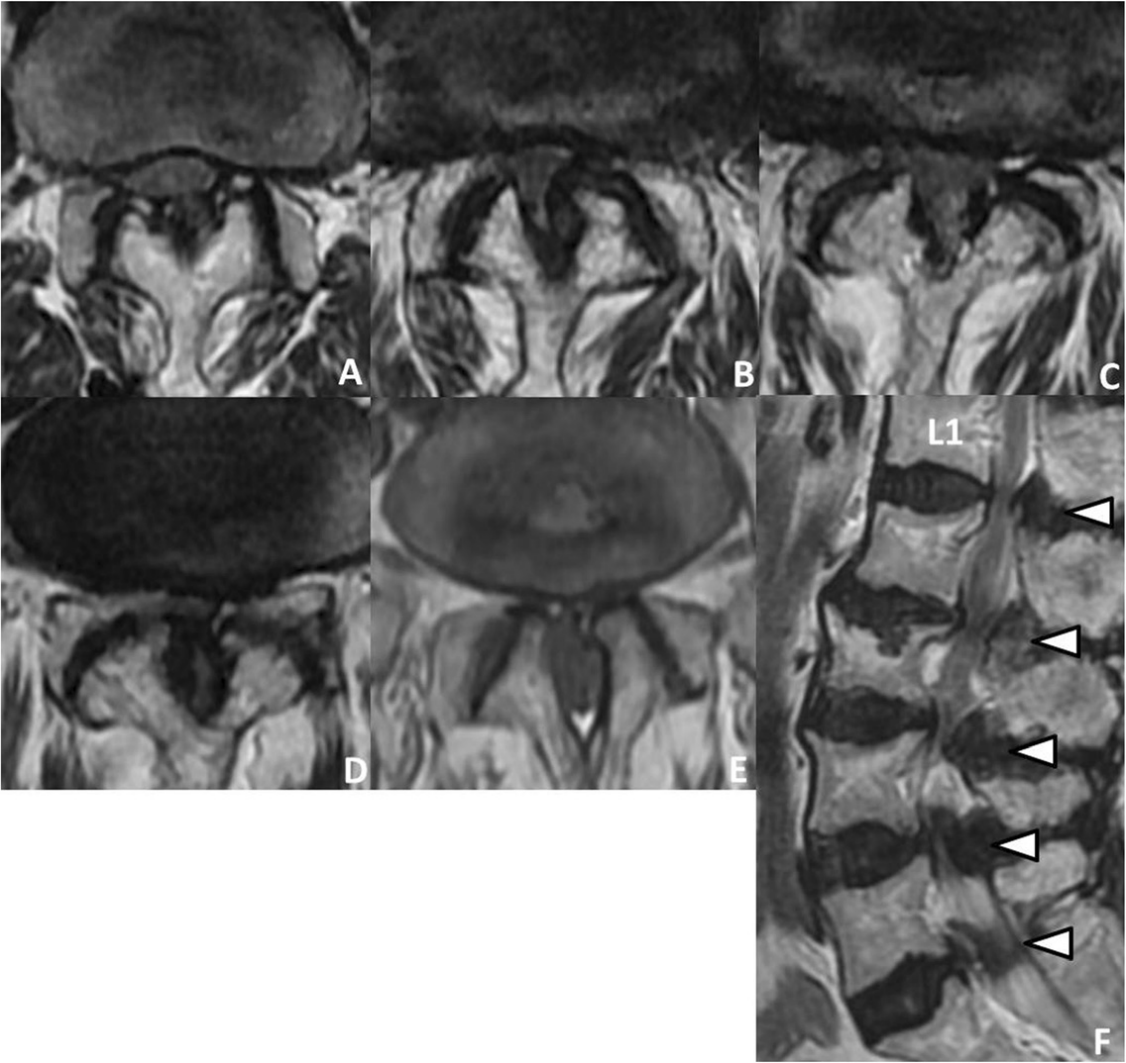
Preoperative magnetic resonance images. (A) through (E) Preoperative magnetic resonance imaging scans show extensive spinal stenosis from L1/2 (A), L2/3 (B), L3/4 (C), L4/5 (D) and L5/S1 (E). (F) Sagittal T2-weighted image reveals multiple stenosis at L1/2-L5/S (arrowheads).
